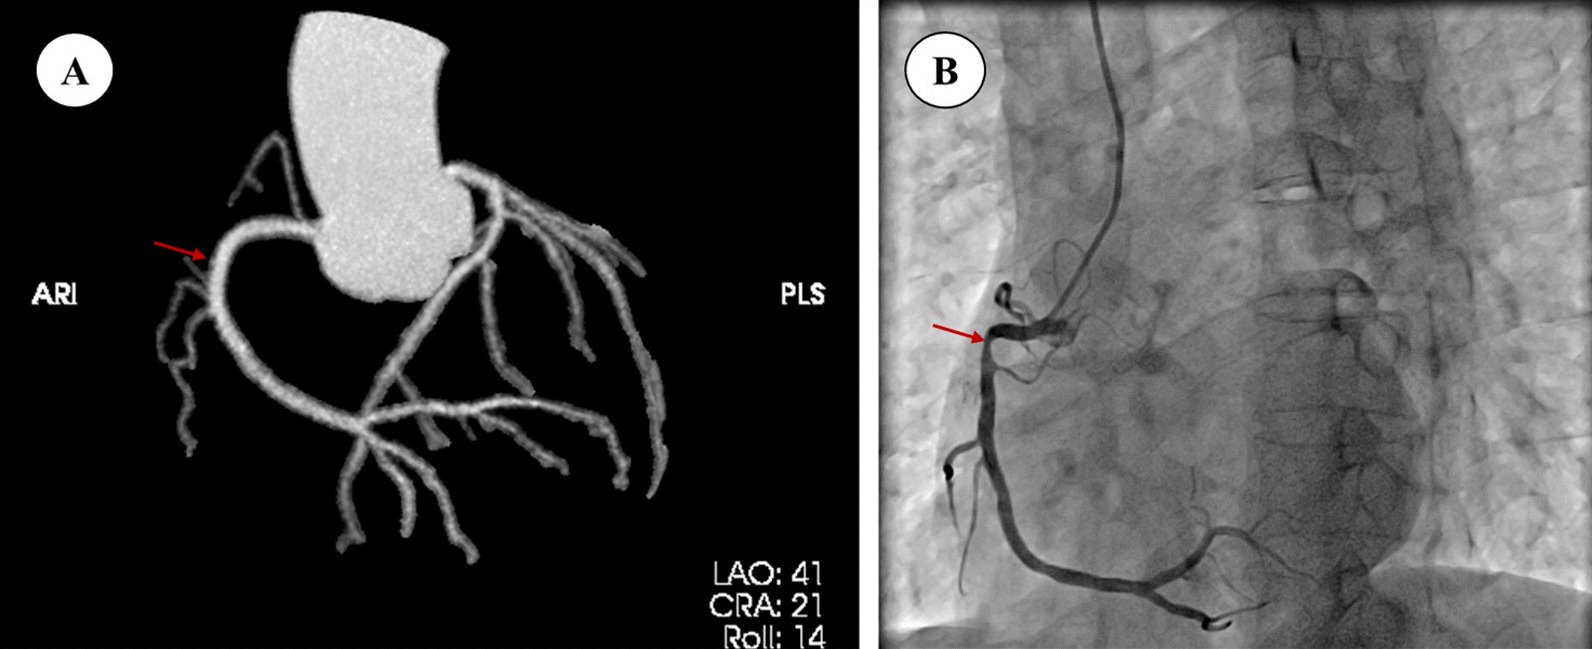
A two years ago, coronary artery CTA showed the right coronary was almost normal. B two years later, coronary angiography showed 80% stenosis (red arrow) of the proximal right coronary artery
